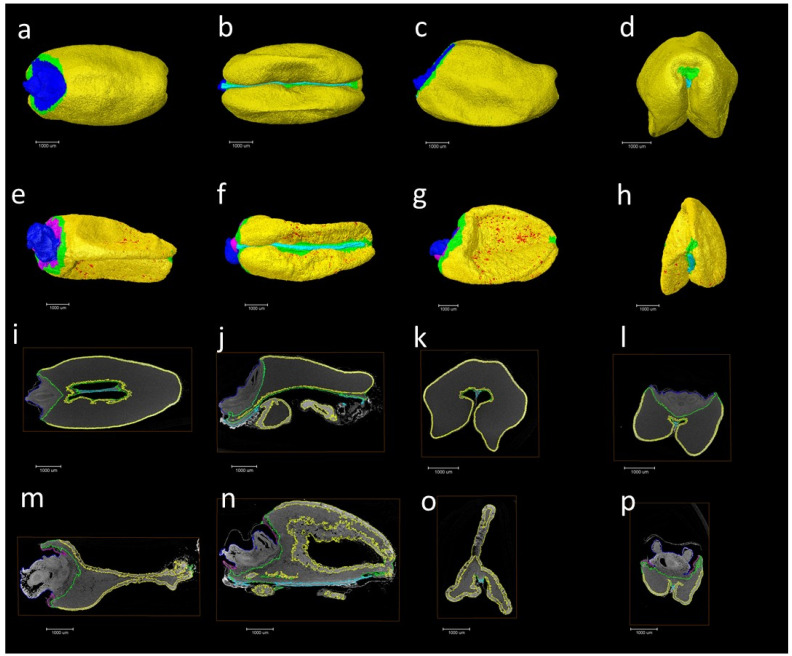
Pictures of seed surfaces (a–h) and examples of seed interiors (i–p) from X-ray microtomography analysis of mature wheat grains from field grown *AsWRI1*-wheat. Pictures show the surface from dorsal side (a,e), ventral side (b,f), lateral side (c,g), and front (d,h) of seeds. Pictures (i–p) show examples of interior parts of seeds from similar orientations. Grain from control plant (line R3P8.12, null) in (a–d,i–l), grain from *AsWRI1*-expressing line (R3P8.10, homozygous with 12 gene inserts) in (e–h,m–p). Color key: Yellow is aleurone, blue is germ and scutellum, green is endosperm, red is air pockets, cyan is the transfer bundle, and violet is the depleted region. Scale bars are 1000 μm.
